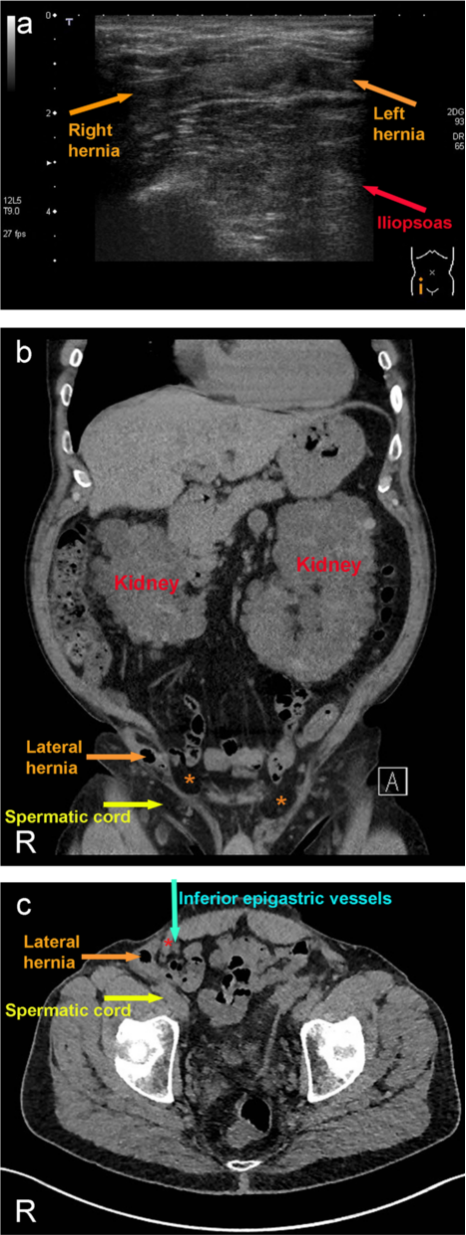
Pre-operative localization of the hernia using (a) ultrasound and (b,c) CT. (a) Hernia on the right side (orange arrow) movable, atypically lateral position. Red arrow points to the iliopsoas muscle. (b) Coronal CT scan demonstrates direct inguinal hernias on both sides (orange asterisks) within the transversal fascia as well as the very lateral inguinal hernia with adipose tissue and small intestine (orange arrow) lateral to the spermatic cord (yellow arrow) on the right side. Note enlarged polycystic kidneys. (c) Horizontal CT scan reveals the position of the lateral hernia (orange arrow) latero-dorsal to lateral abdominal wall muscles (red asterisk), in particular internal oblique and transversus abdominalis muscles, to the inferior epigastric vessels (turquoise arrow), and the inguinal ligament, and lateral to the spermatic cord (yellow arrow).
